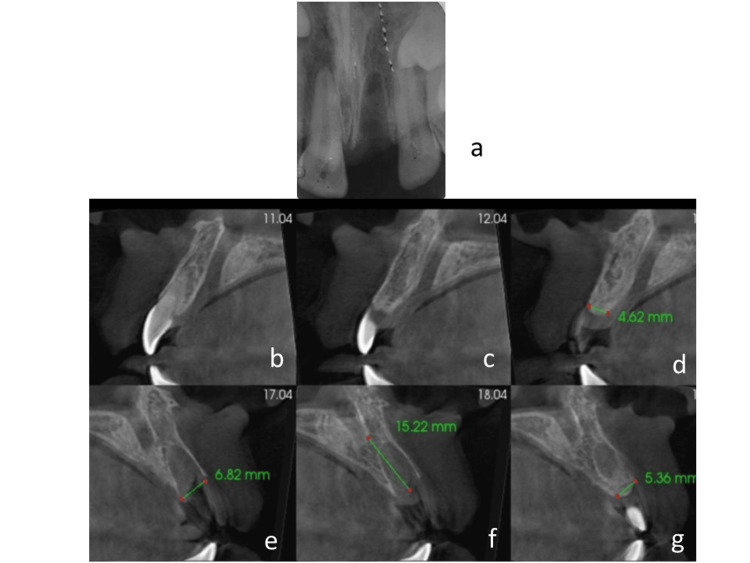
Radiographic investigation (a) Periapical view of the edentulous area;  (b)-(g) CBCT of the cross-sectional view of intact buccal and lingual bone plate showing the thickness of available bone CBCT: cone-beam computed tomography
